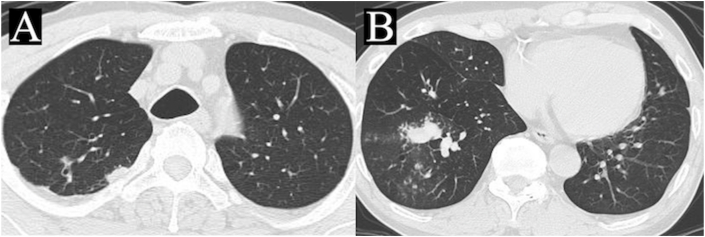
At the first visit to a primary care doctor, chest CT showed the new infiltrations in the upper (A) and lower lobes (B) of the right lung. CT: computed tomography.
